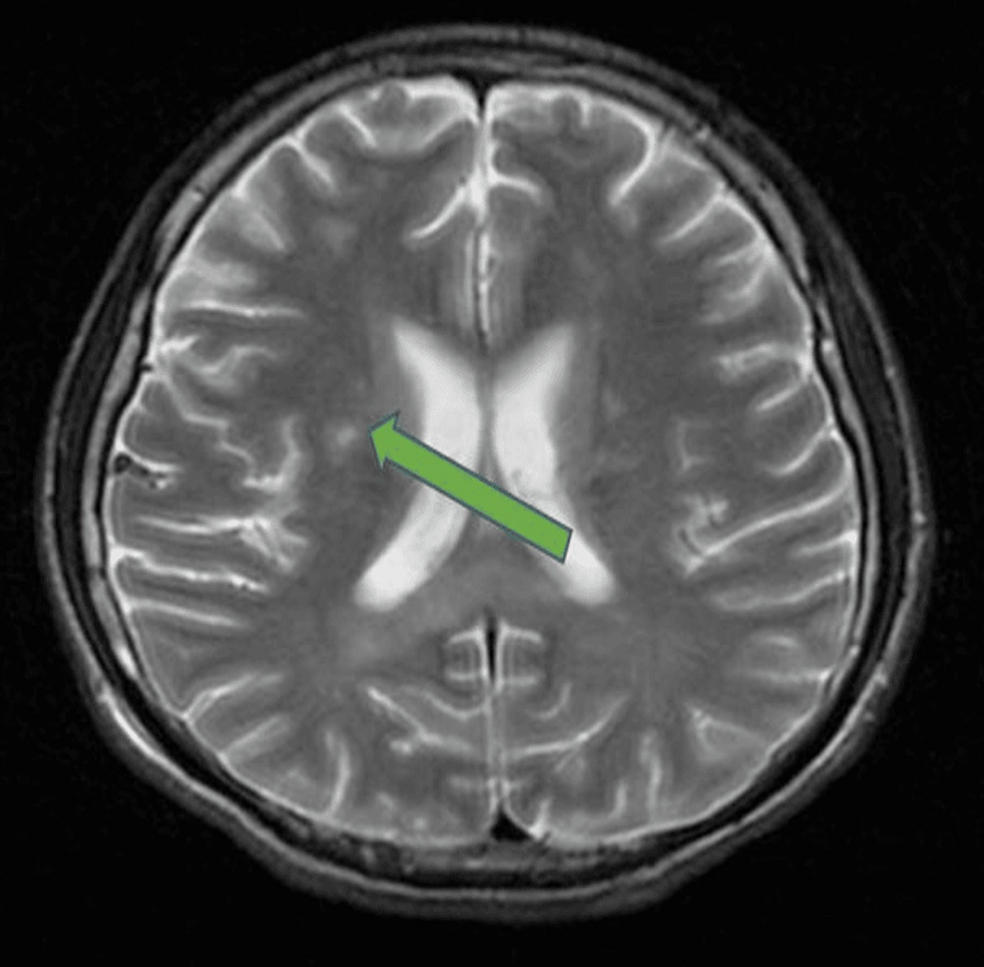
T2 weighted image brain MRI showing patchy ill-defined hyperintensities throughout the frontoparietal lobes, involving the subcortical, periventricular and deep white matter region
